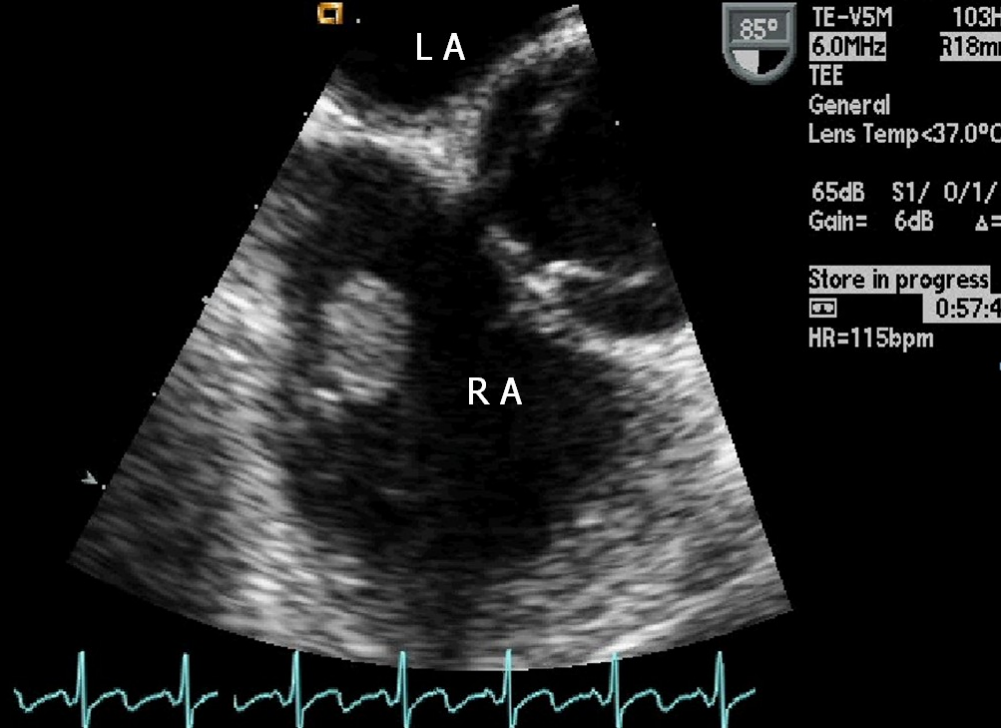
Transesophageal echocardiography at 85° view showing a mass with a stalk in right atrium. LA = left atrium; RA = right atrium.
